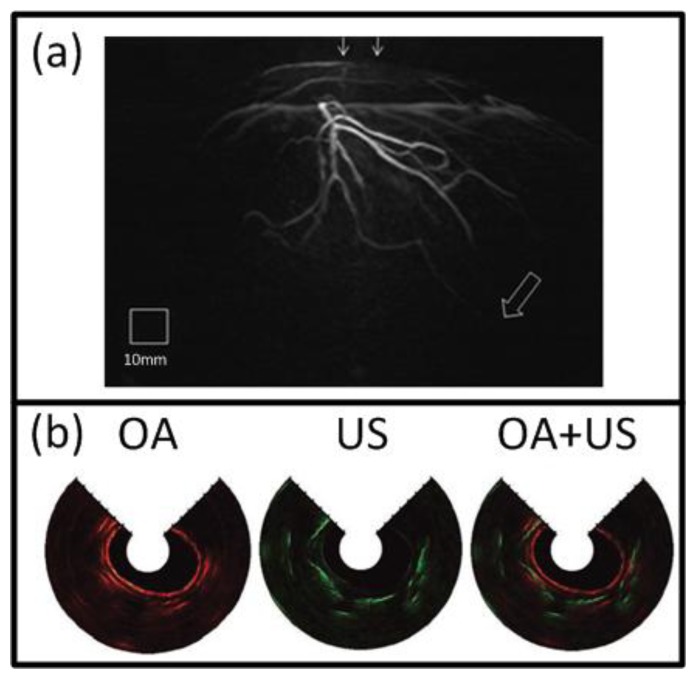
(a) OA angiography of the breast by Kruger *et al*. without contrast enhancement [49]. Maximum intensity projection of a 57 year old volunteer's breast showing the vasculature from superficial areas (solid arrows) up to considerable depth (hollow arrow). *Reprinted with permission from American Association of Physicists in Medicine*; (b) Simultaneous, dual-wavelength OA and US endoscopy by Yang *et al*. [47]. Ultrasonic, optoacoustic and combined cross-sectional *in vivo* images of a rabbit esophagus near the lungs taken at 584 nm. *Reprinted with permission from Nature Publishing Group*.
